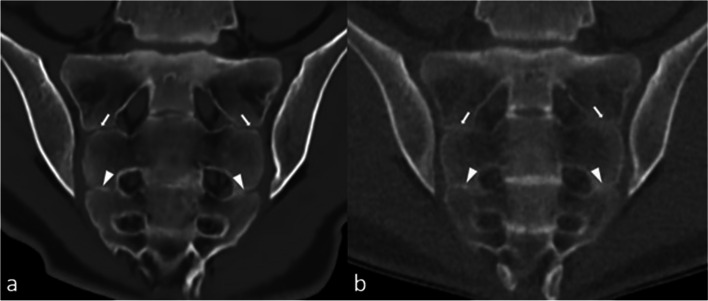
Comparison between MRI-based synthetic CT (sCT) (a) and CT (b) in a 13-year-old boy in a paracoronal plane. Arrows indicate open growth plates at the levels S1–S2 and arrowheads indicate partially closed growth plates at the S2–S3 level
